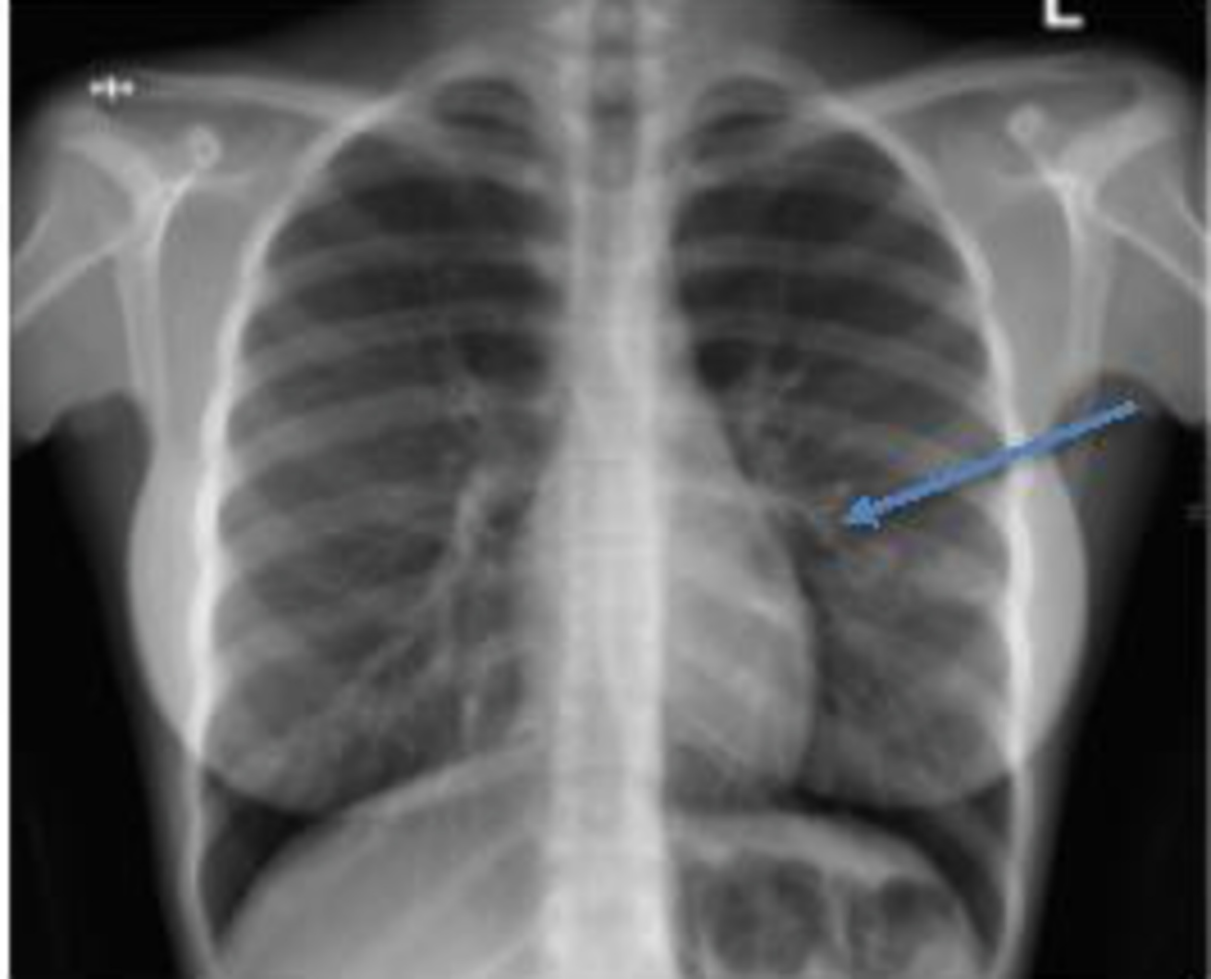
Chest X-ray showing nonhomogeneous cystic and nodular infiltrates at the left mid zone.
